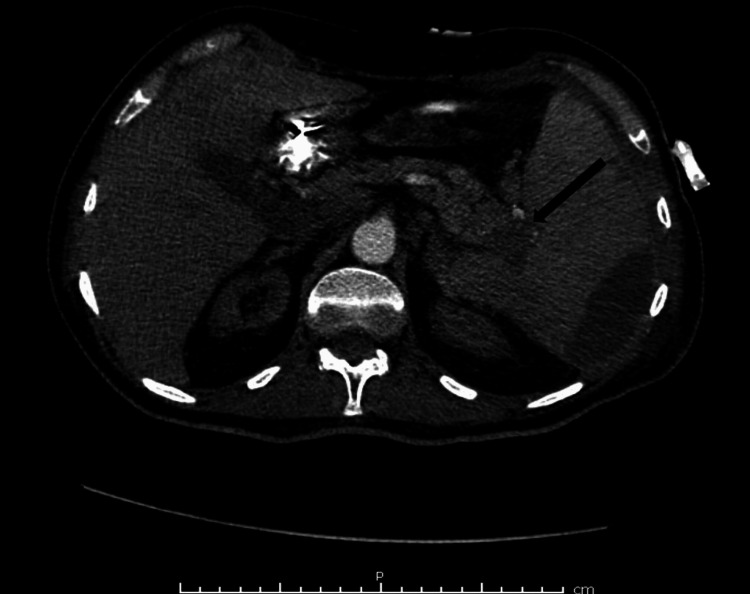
Repeat computed tomography scan of the abdomen showing hypoattenuated area in the tail of the pancreas (arrow).
